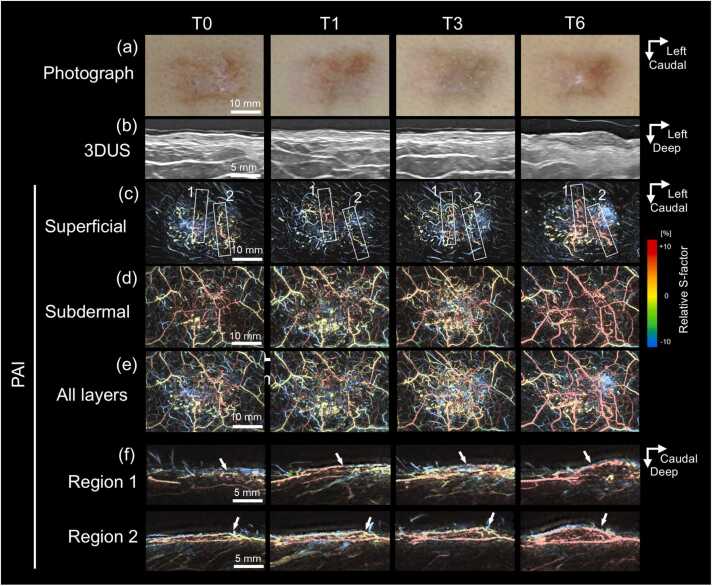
Longitudinal changes in a recurrent Case 4. Before treatment, the keloid exhibited a flat, oval morphology and subsequently recurred between T3 and T6 (a). Ultrasound images demonstrate thickening of the keloid profile at recurrence (b). Frontal-view photoacoustic images depict blood vessels and their relative S-factor using a color gradient across tissue layers (c–e). Aberrant tortuous microvessels are observed in the superficial layer (c). The subdermal layer contains a vascular network with heterogeneously elevated oxygen saturation (d, e). Sectional photoacoustic images (f), corresponding to rectangular regions 1 and 2 in (c), show upward displacement of superficial microvessels as the lesion thickened, with these vessels eventually overlying the recurrent lesion (arrows). At T6, the superficial microvessels exhibit increased oxygen saturation and demonstrate peripheral continuity with the subdermal vascular network.
